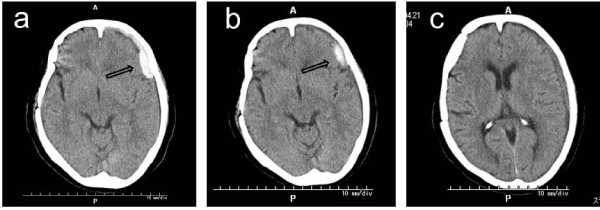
CT findings. (A) Immediately after onset, a subdural hematoma is observed extending from the left frontal to temporal region (arrow). (B) On day 12 after onset, the volume of the hematoma is reduced and density is lowered (arrow). (C) One month after onset, further reduction in the volume of the hematoma is observed.
